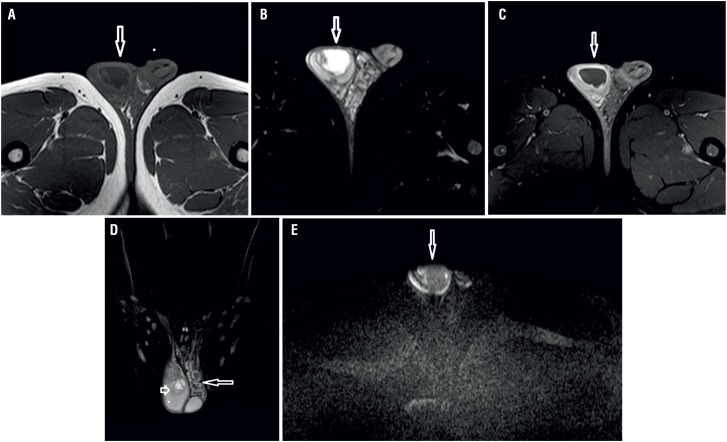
A 26-year-old male patient. On T1 weighted image (a) a hypointense cystic lesion with a thick wall is seen within the right testicle. The lesion appears hyperintense (arrow) on T2-weighted image (b). Lesion's wall shows marked enhancement after intravenous gadolinium injection (arrow) (c). Coronal T2 weighted image (d) shows the same lesion and contralateral varicocele. on diffusion weighted image obtained with a b value of 1000s/mm2 (e), the lesion shows restricted diffusion and appears hyperintense (arrow).
